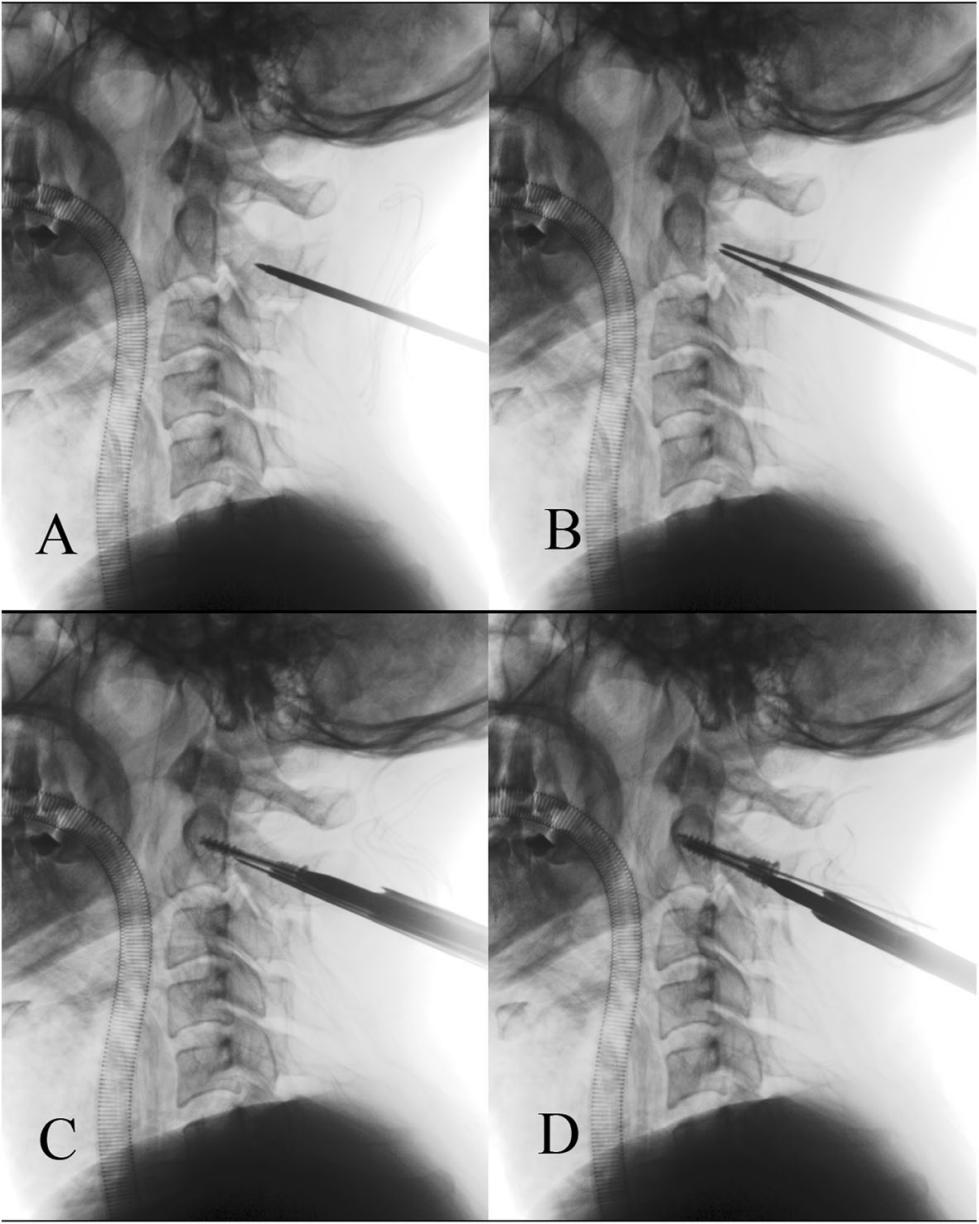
Intraoperative images of C-arm radiographs. a Confirm the entry point and the direction of the screw. b Insert the guide pin through the fracture line and then drill a hole by the drill bit along the direction of the guide pin. c, d Screw appropriate-sized new transpedicular lag screws on either side by passing through the drill hole and achieve firm compression and fixation
